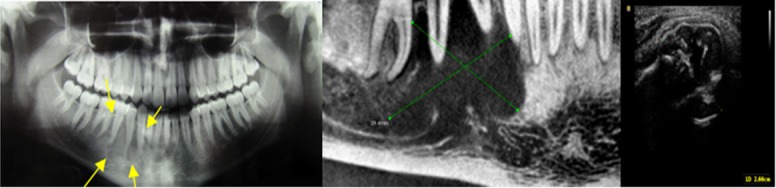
A case of central giant cell granuloma. (A) Panoramic view reveals radiolucent lesion with scalloped margin in the right mandibular body. (B) CBCT shows lytic lesion in right mandibular body. (C) Ultrasound image shows mixed echogenicity (hyperechoic-hypoechoic pattern)
